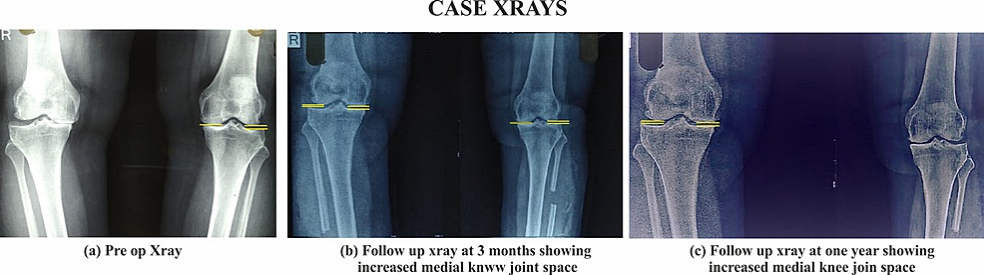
Images showing preoperative and postoperative X-rays
